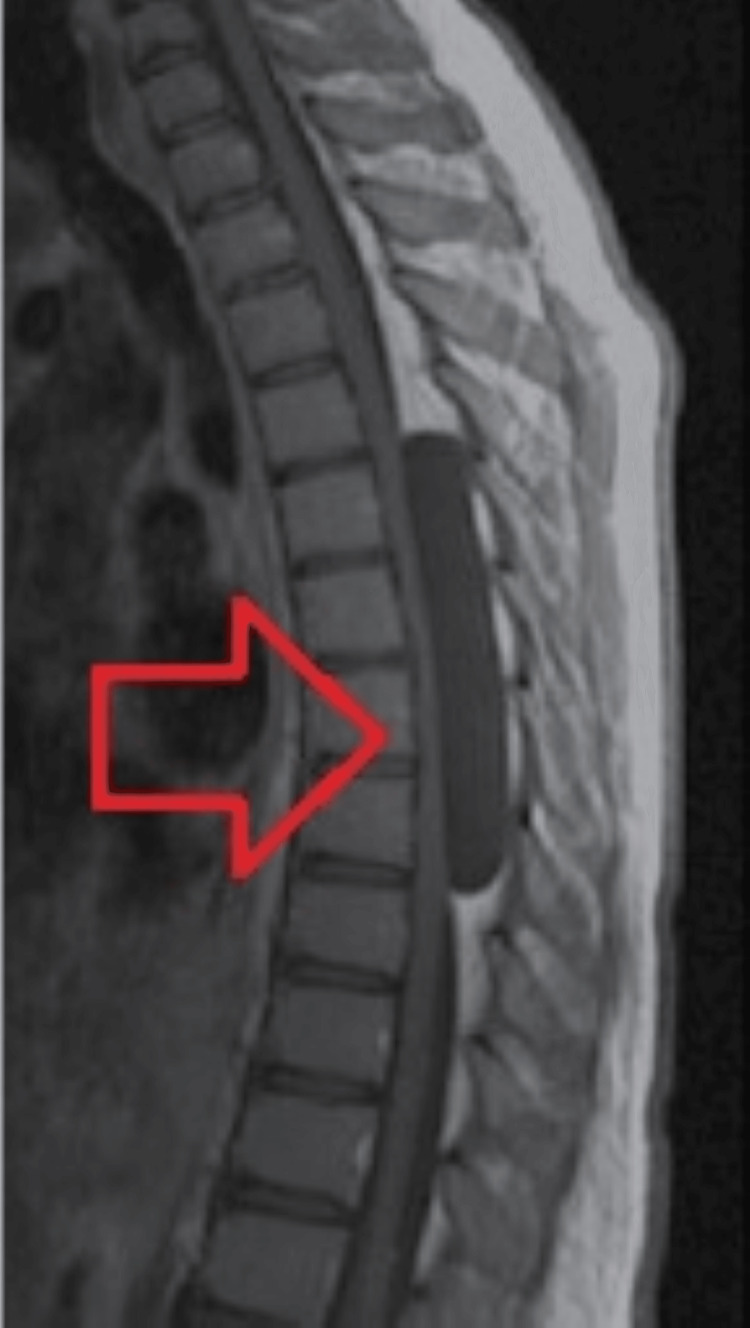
Thoracic T1-weighted MRI showing an extradural cyst MRI: magnetic resonance imaging
